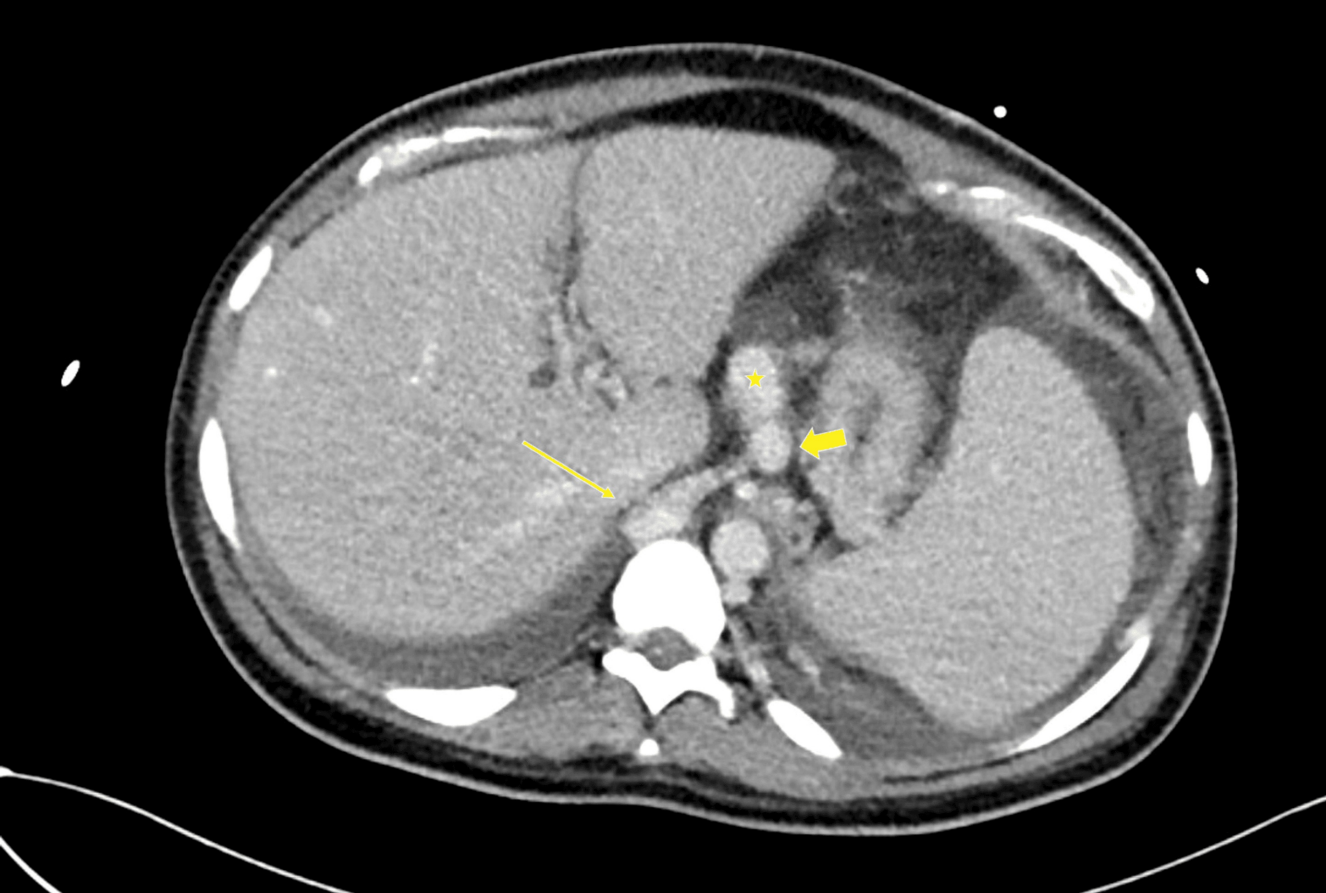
Axial CT scan showing the Abernethy malformation type Ib Thin arrow: inferior vena cava; thick arrow: splenic vein; asterisk: superior mesenteric vein
